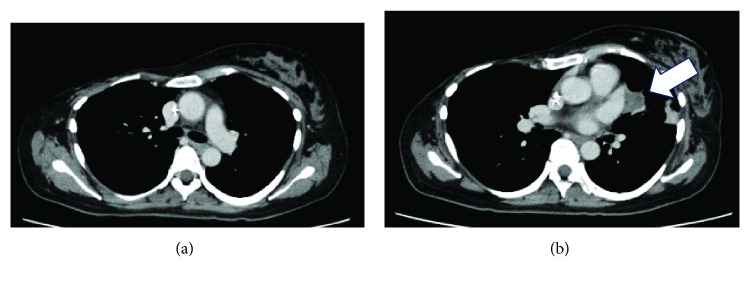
Computed tomography scan acquired after doxorubicin-ifosfamide (AI) chemotherapy showing (a) complete regression of the right lung mass and (b) partial regression of the left lung mass to 2 cm (arrow) and an absence of pleural effusion.
